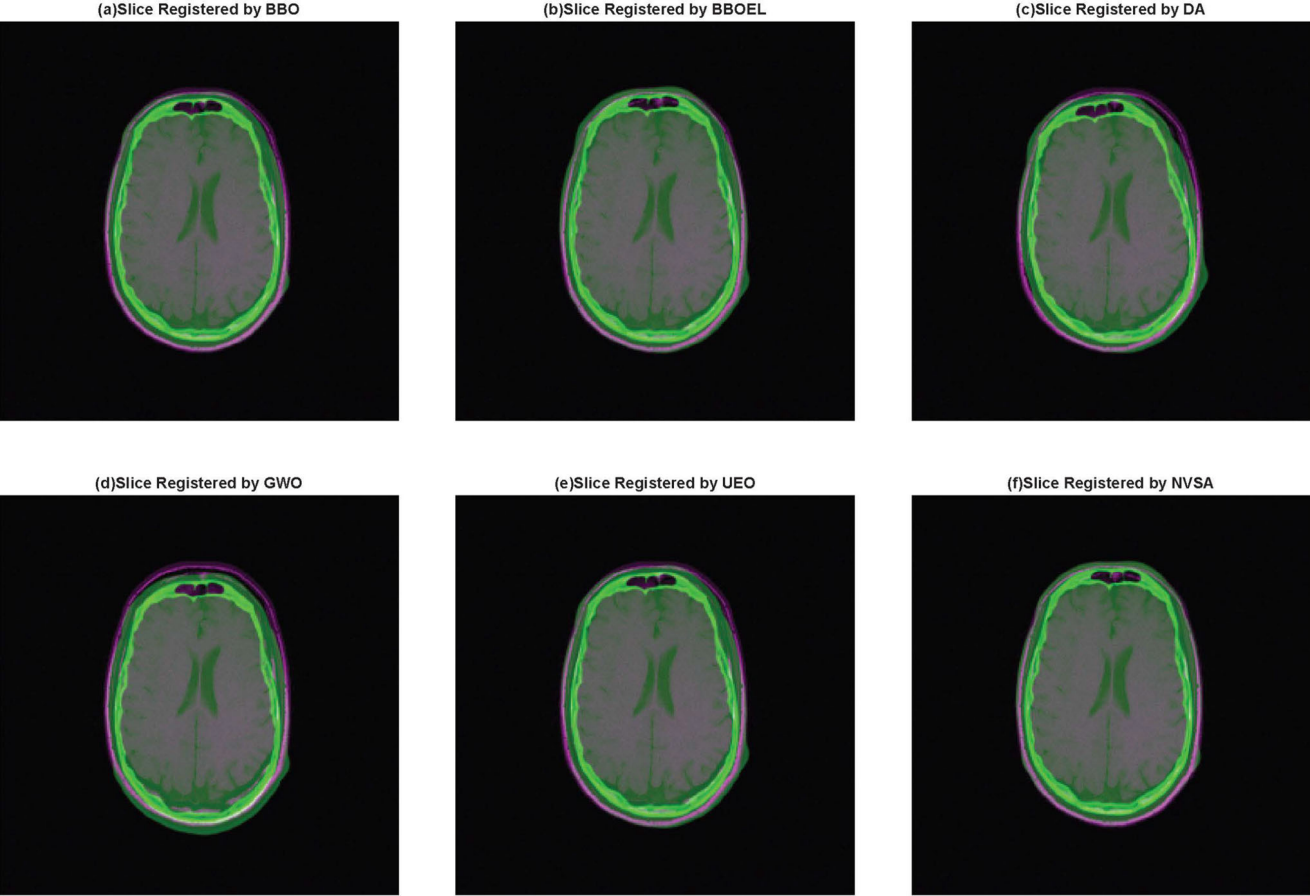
Visualizations of the registration process between multimodal P002 CT-T1 image by using six different metaheuristic algorithms
